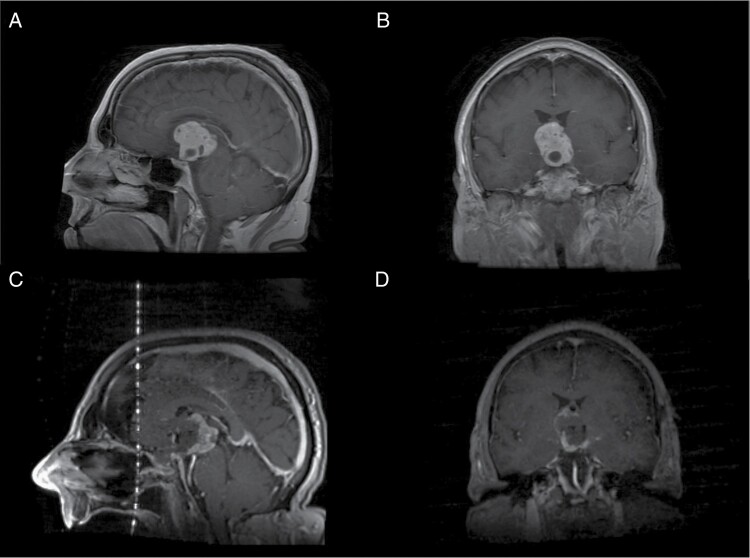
Preoperative and intraoperative MRI of the suprasellar lesion. Preoperative T1-weighted post-contrast MRI in the (A) sagittal and (B) coronal views demonstrate a contrast-enhancing lesion, measuring 3.8 × 3.0 × 3.5 cm. After subtotal resection via an endoscopic endonasal approach, intraoperative T1-weighted post-contrast MRI in the (C) sagittal and (D) coronal views demonstrate expected residual enhancing tumor left along the posterior aspect of the capsule, adherent to the hypothalamus.
